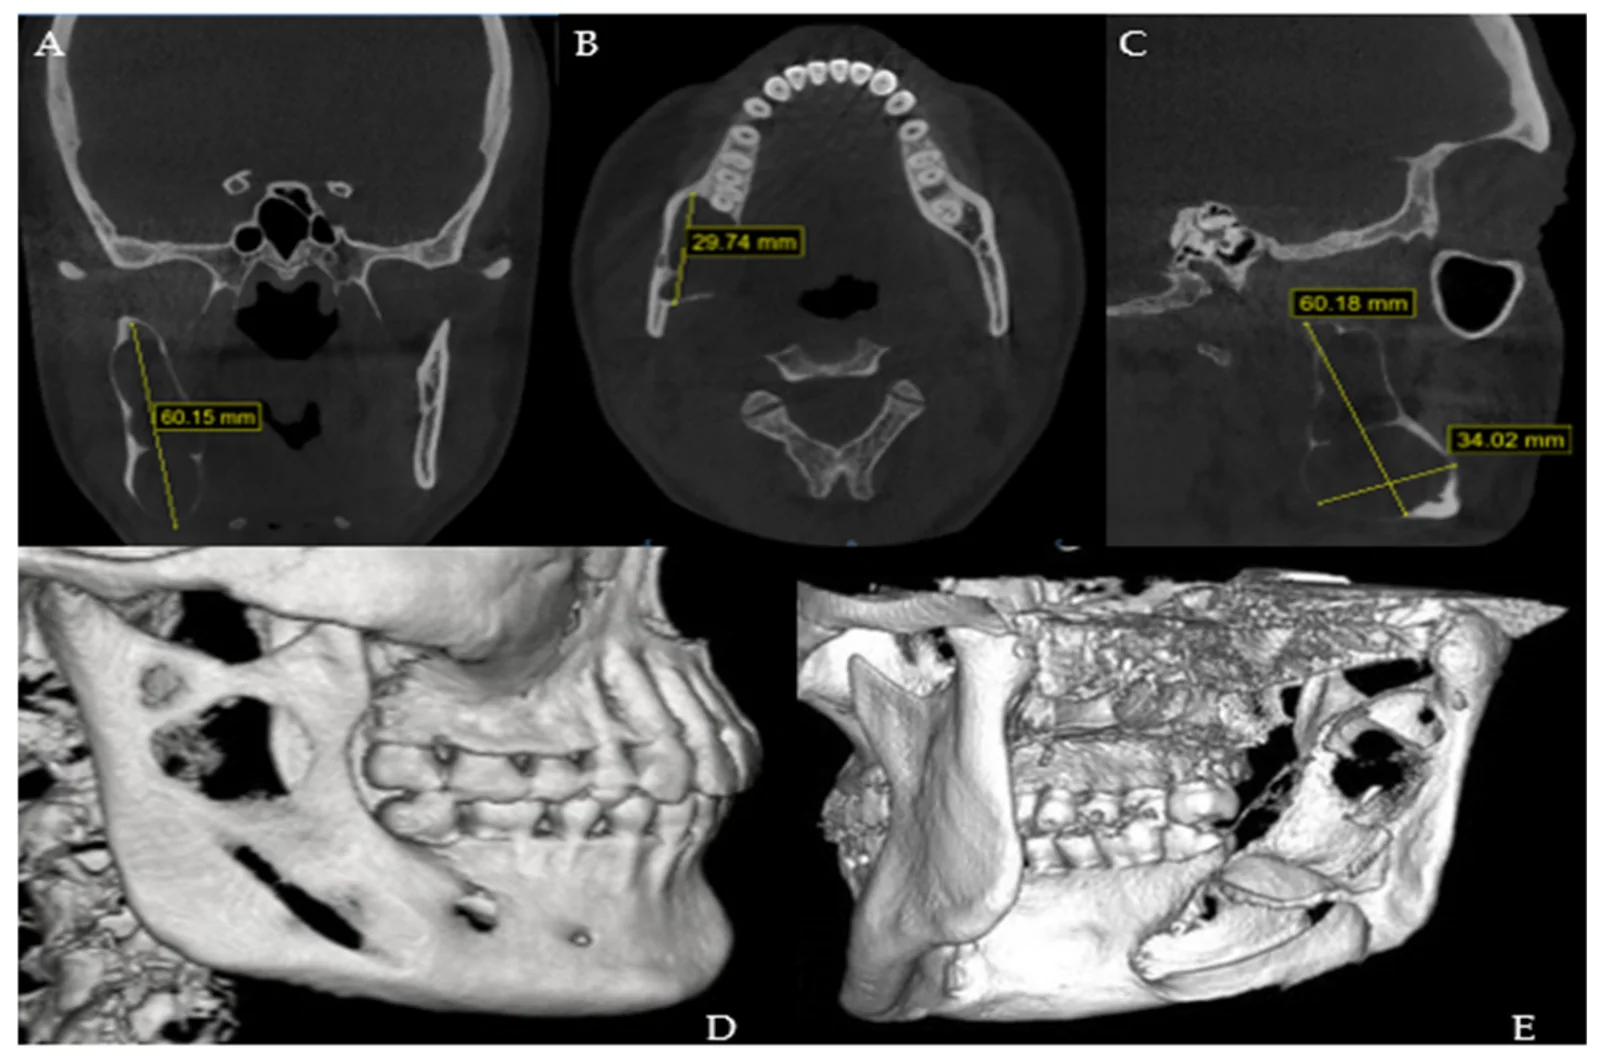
Initial cone-beam computed tomography (CBCT). (A) Coronal, (B) axial, and (C) sagittal sections; (D) right lateral and (E) left posterior oblique views of the mandibular tomographic reconstruction. The lesion appears as a well-defined hypodense area without cortical perforation or root resorption. Yellow measurement lines indicate the initial lesion dimensions (approximately 6 × 3.4 × 2.9 cm).
